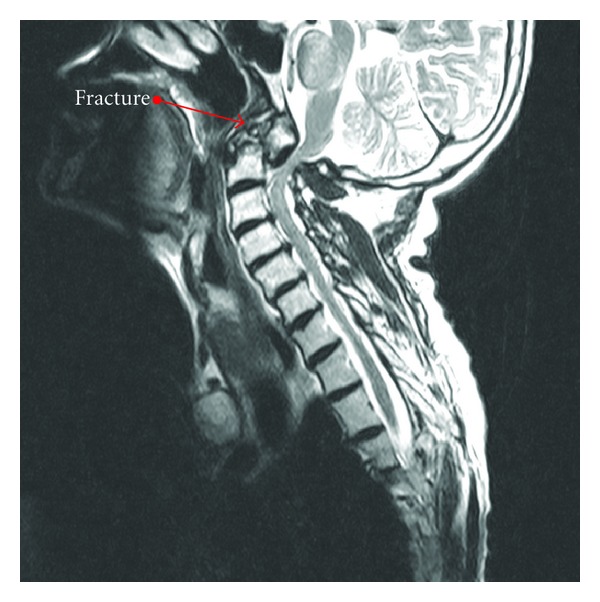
It shows a contrasted MRI with cervical spine fracture.
